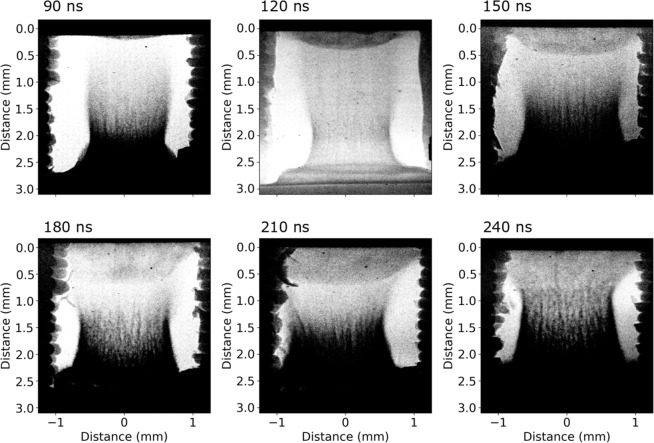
X-ray radiography images of plasma flow and reverse shock at different time delays. The laser driver interacts with the target at the bottom of the image creating a plasma flow moving upwards. The reverse shock initially moves downward away from the obstacle before reaching a stationary position beginning at 180 ns. The width of the reverse shock increases with time, indicating the presence of mass being evacuated in the lateral directions. The structures on the side of the images are not in the same plane as the experiment and hence do not play any role in the dynamics. Further details can be found in the Methods section.
